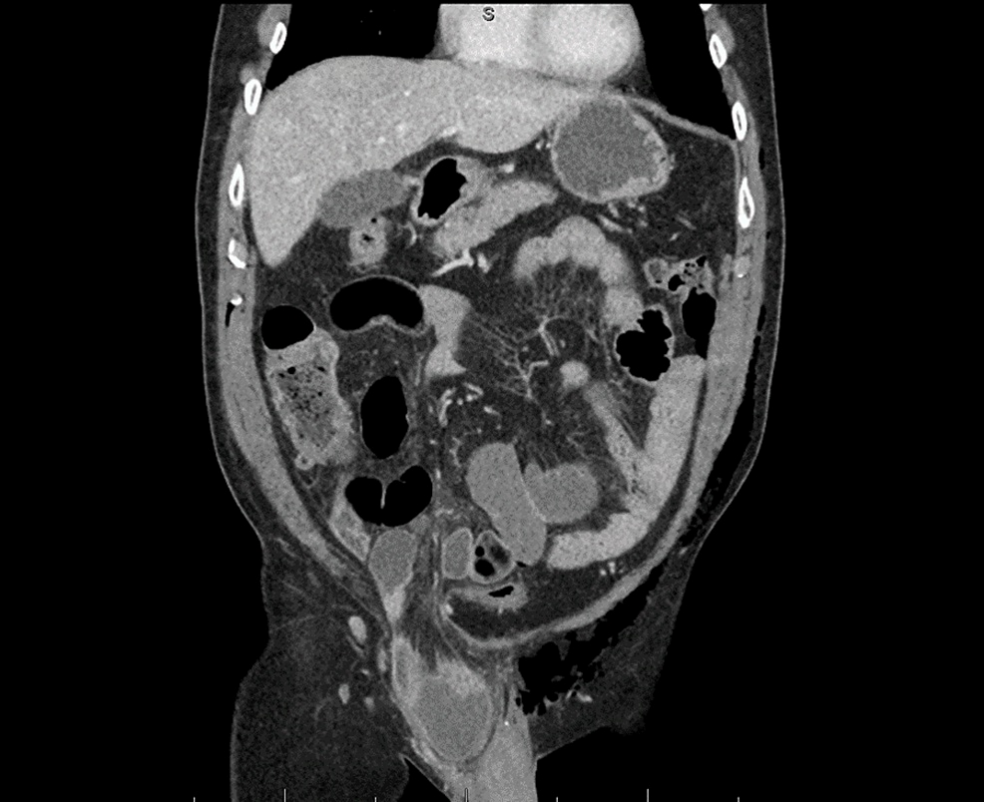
CT showing small bowel incarceration of the right inguinal hernia containing small bowel now with fat stranding and a dilated, fluid-filled loop of the small bowel, with associated upstream intra-abdominal small bowel dilatation and gas fluid levels, suggesting small bowel obstruction CT: computed tomography
